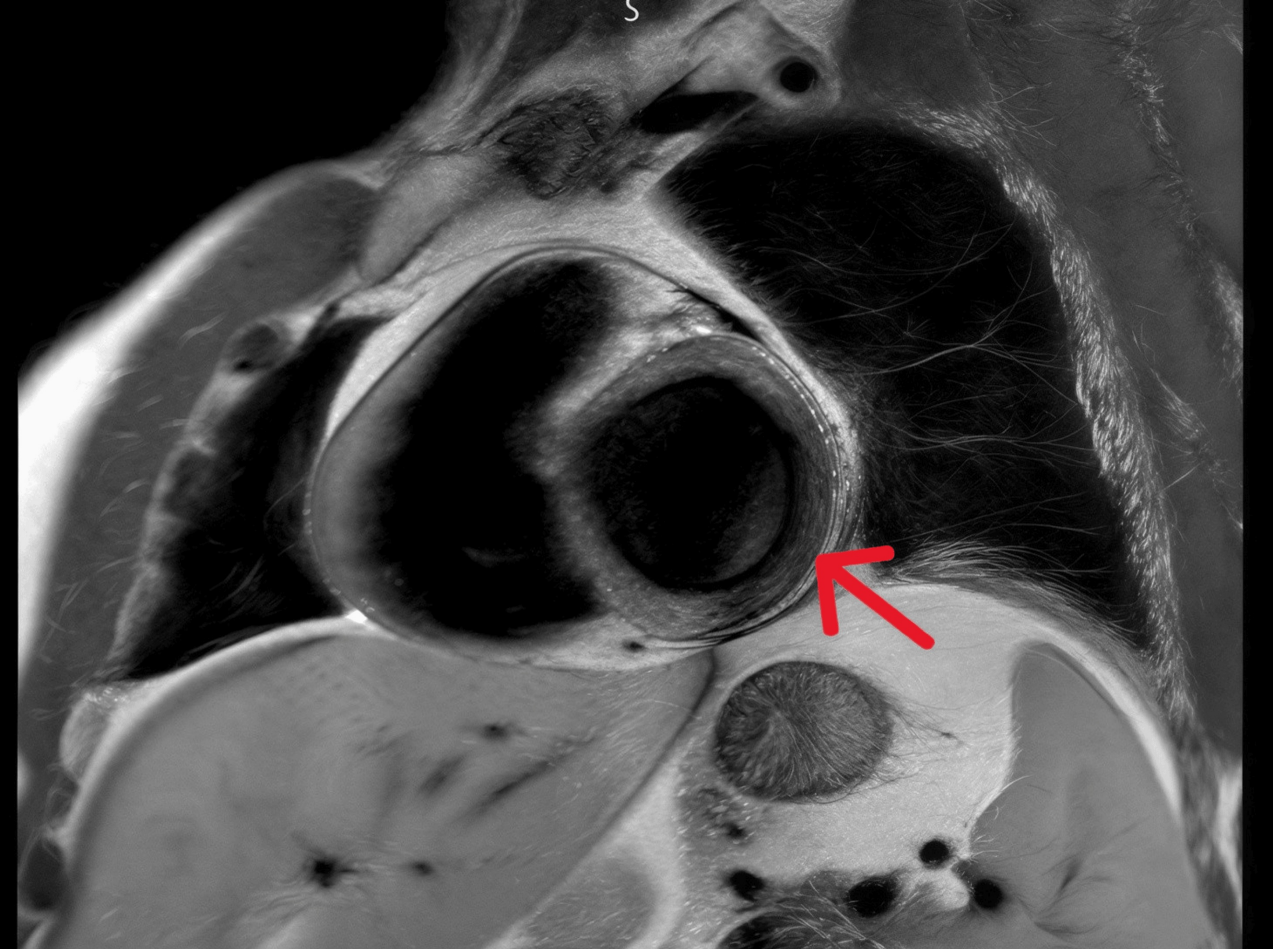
Cardiac magnetic resonance image The arrow indicates patchy myocardial edema with subepicardial early and late gadolinium enhancement involving the lateral and inferior left ventricular walls, a pattern that is typical of inflammatory myocardial injury, consistent with acute myocarditis.
